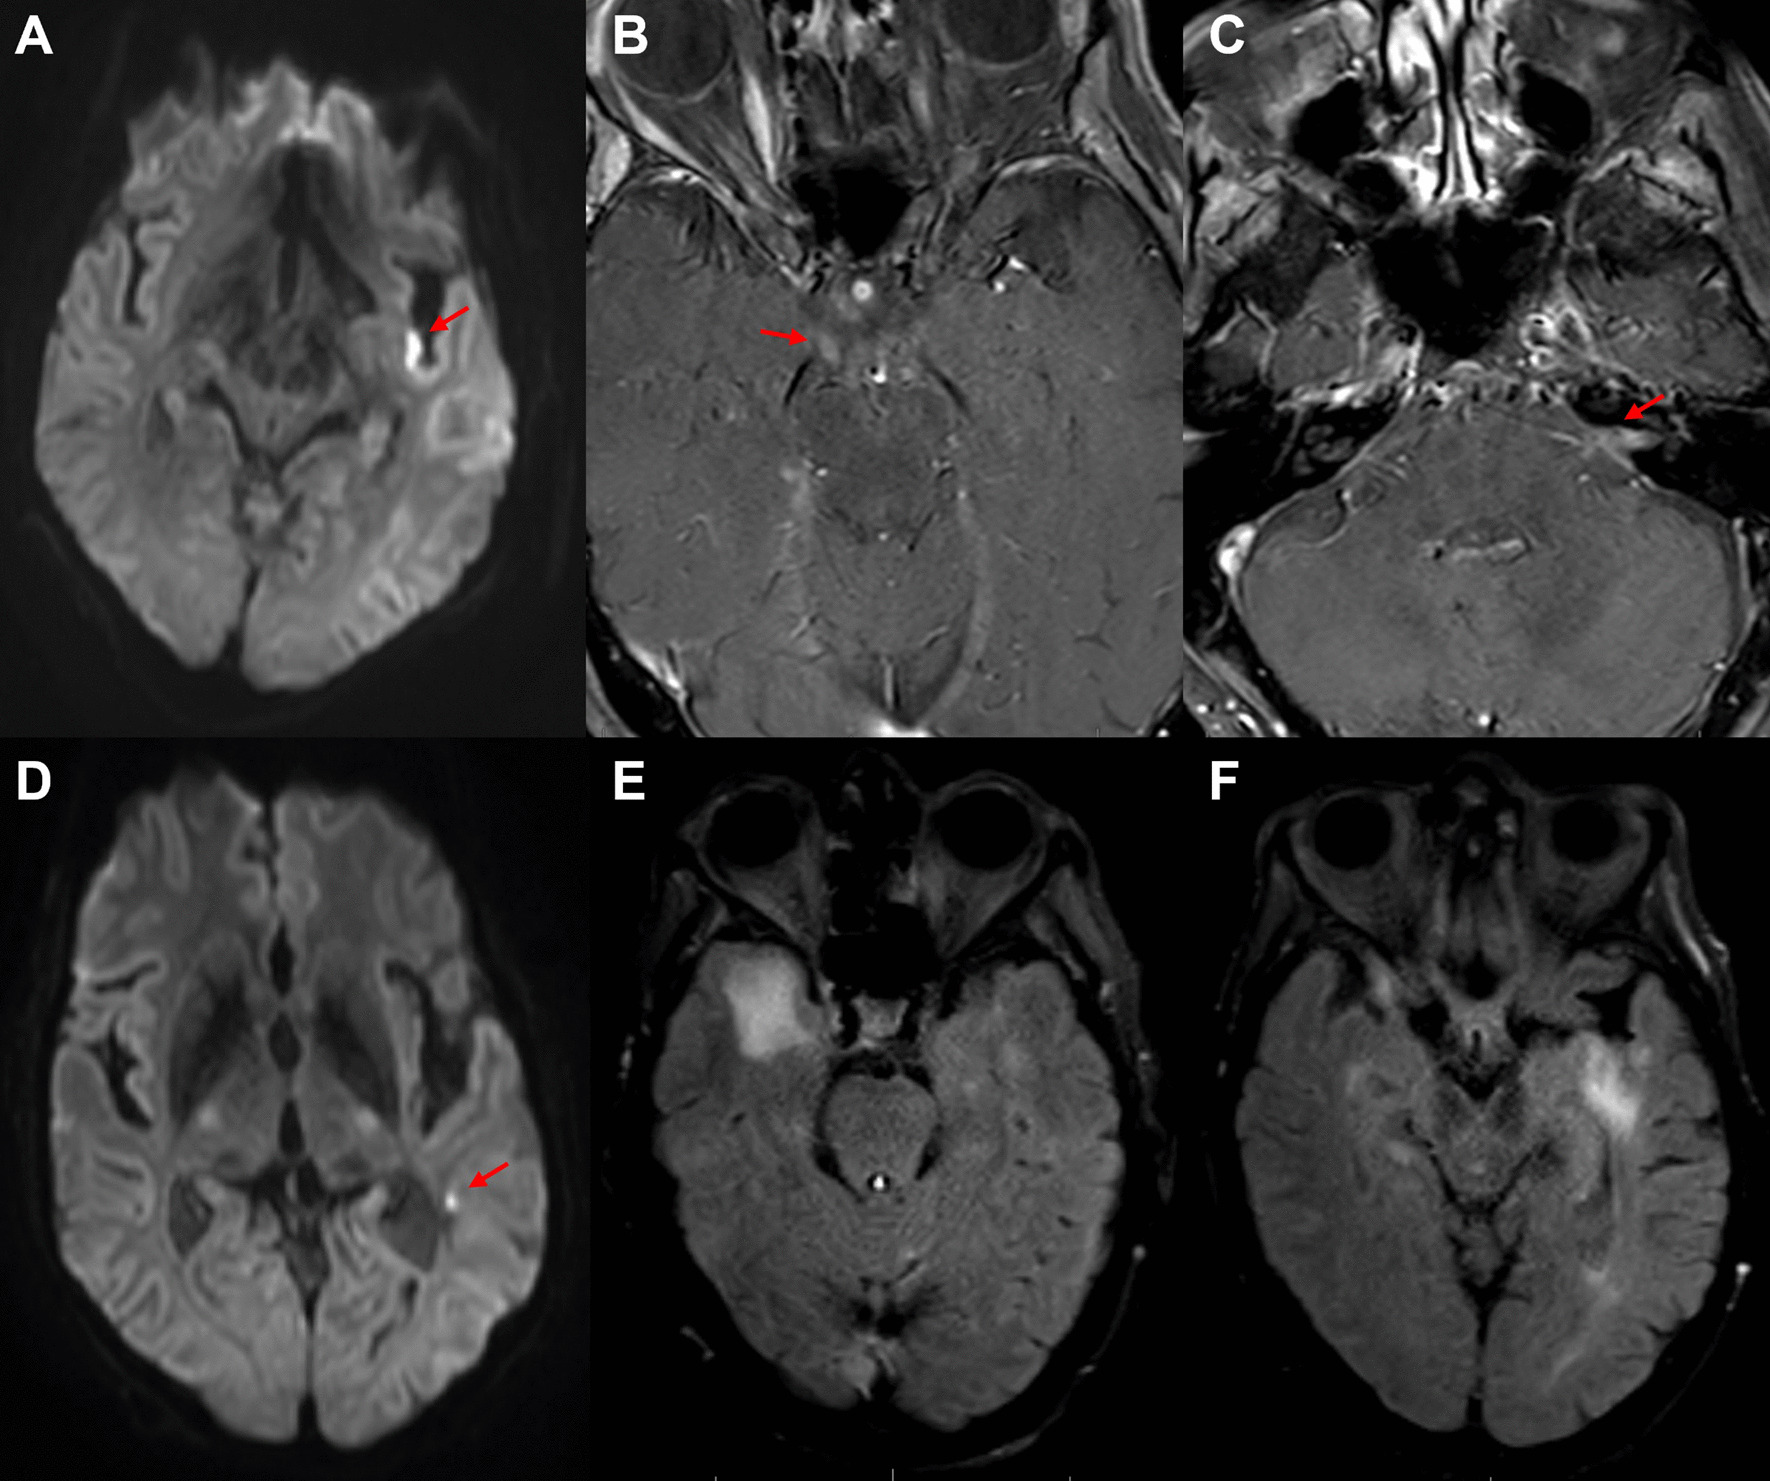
EBV encephalitis. MRI of the brain revealed a small area of diffusion restriction along the posterior left insula, consistent with an acute infarct (A); multiple cranial enhancement including the right oculomotor nerve (B, arrow), left facial and vestibulocochlear nerve complex (C, arrow), and left trigeminal nerve (not pictured). There were also new areas of restricted diffusion in the left frontal operculum and posterior left temporal lobe white matter (D, arrow). Two weeks later, MRI of the brain showed multiple areas of non-enhancing FLAIR hyperintensity in the bilateral temporal lobes (E, F)
